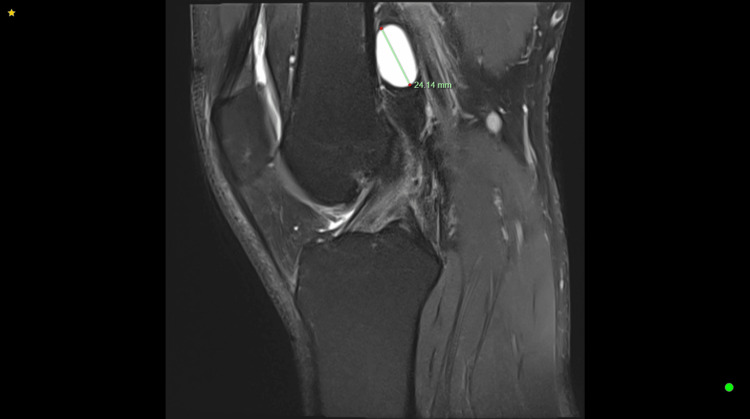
T2-weighted MRI of the left knee (sagittal view) shows a well-defined ovoid cystic structure measuring 24.14 mm, abutting the posterior cortex of the femur.
